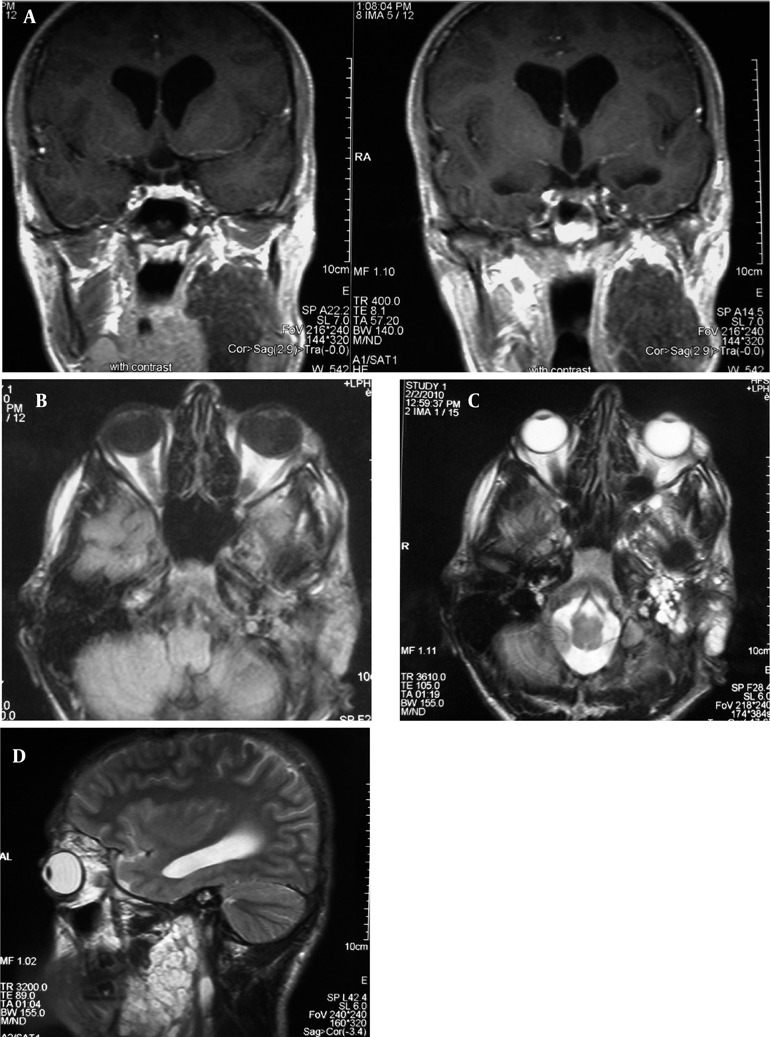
MRI findings of the infratemporal mass A, Coronal T1 weighted MRI (TR/TE = 400/8.1) showing well-defined large mass with homogeneous low signal intensity and mild internal enhancement. Pressure effect on lateral wall of naso- and oropharynx is observed; B, Axial T1 weighted image showing low signal mass adjacent to parotid gland; C and D, Axial and sagittal T2 weighted images (TR/TE = 3610/105) reveal high signal intensity of the mass in the infratemporal fossa and adjacent to the parotid gland.
